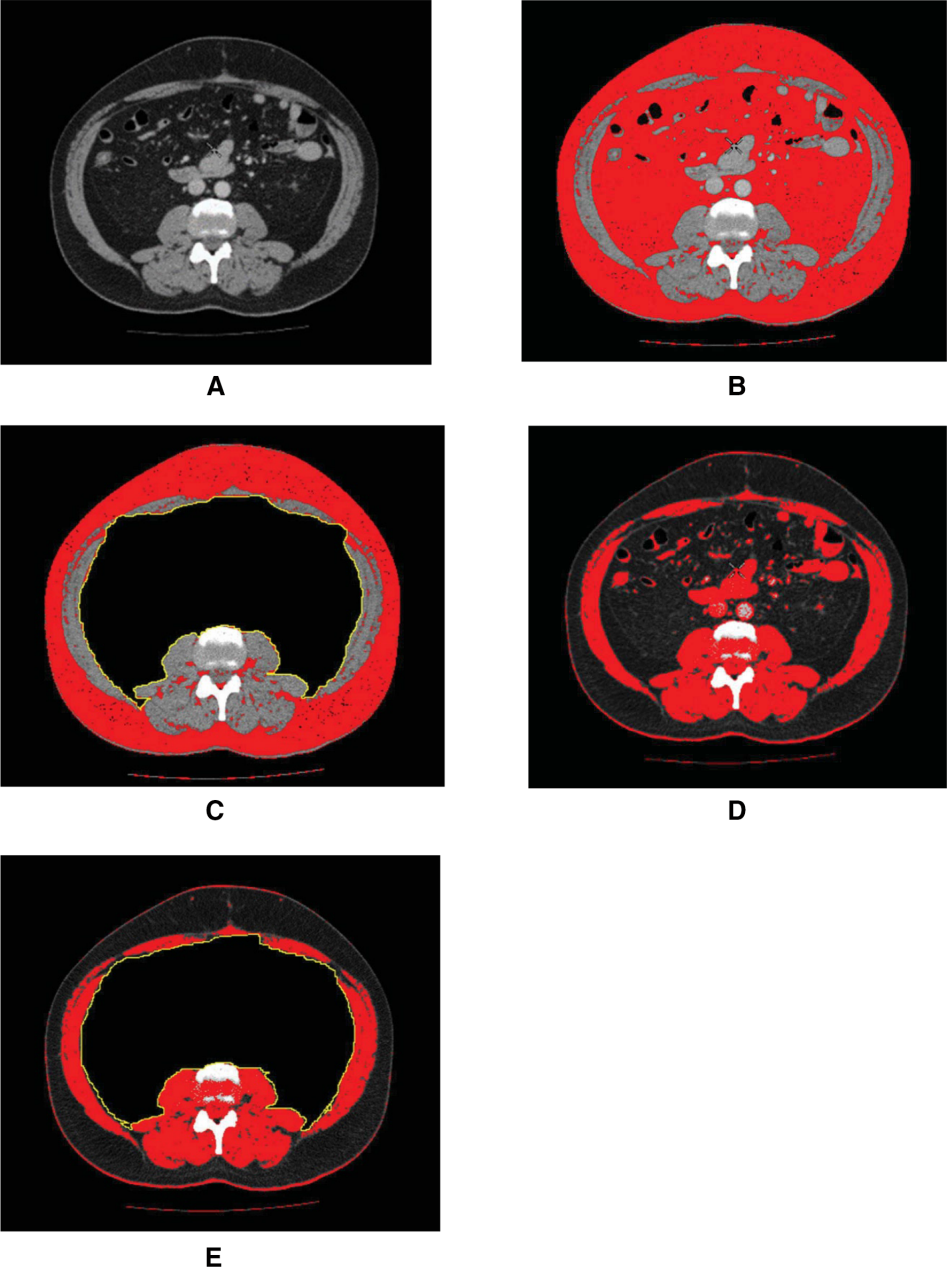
An example of calculating adipose tissue and muscle cross sectional area using J-image software. a Original CT b CT with adipose tissue thresholds applied—adipose tissues appear red. c Abdominal contents cropped, subcutaneous adipose tissue cross sectional area calculated. d Skeletal muscle thresholds applied, muscles appear red. e Abdominal contents cropped; total skeletal, left and right recti muscles cross sectional area calculated
